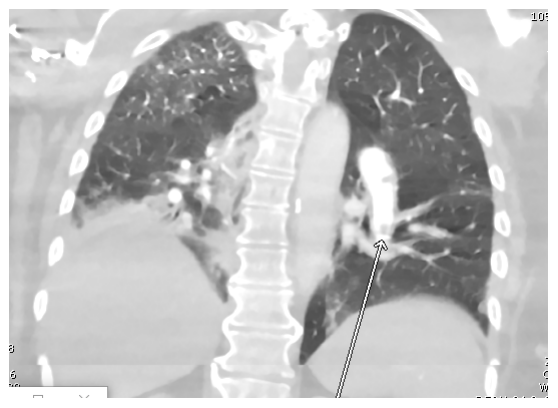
CTA chest showing an embolus in a branch of the left pulmonary artery (arrow).
